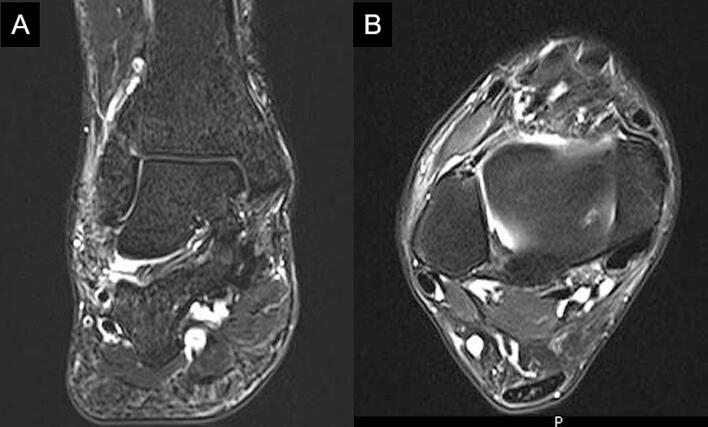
Magnetic resonance imaging of the right ankle shows a rupture of the right calcaneofibular ligament in the fat-suppressed T2-weighted coronal image (A) and an injury of the right anterior talofibular ligament in the fat-suppressed T2-weighted axial image (B).
